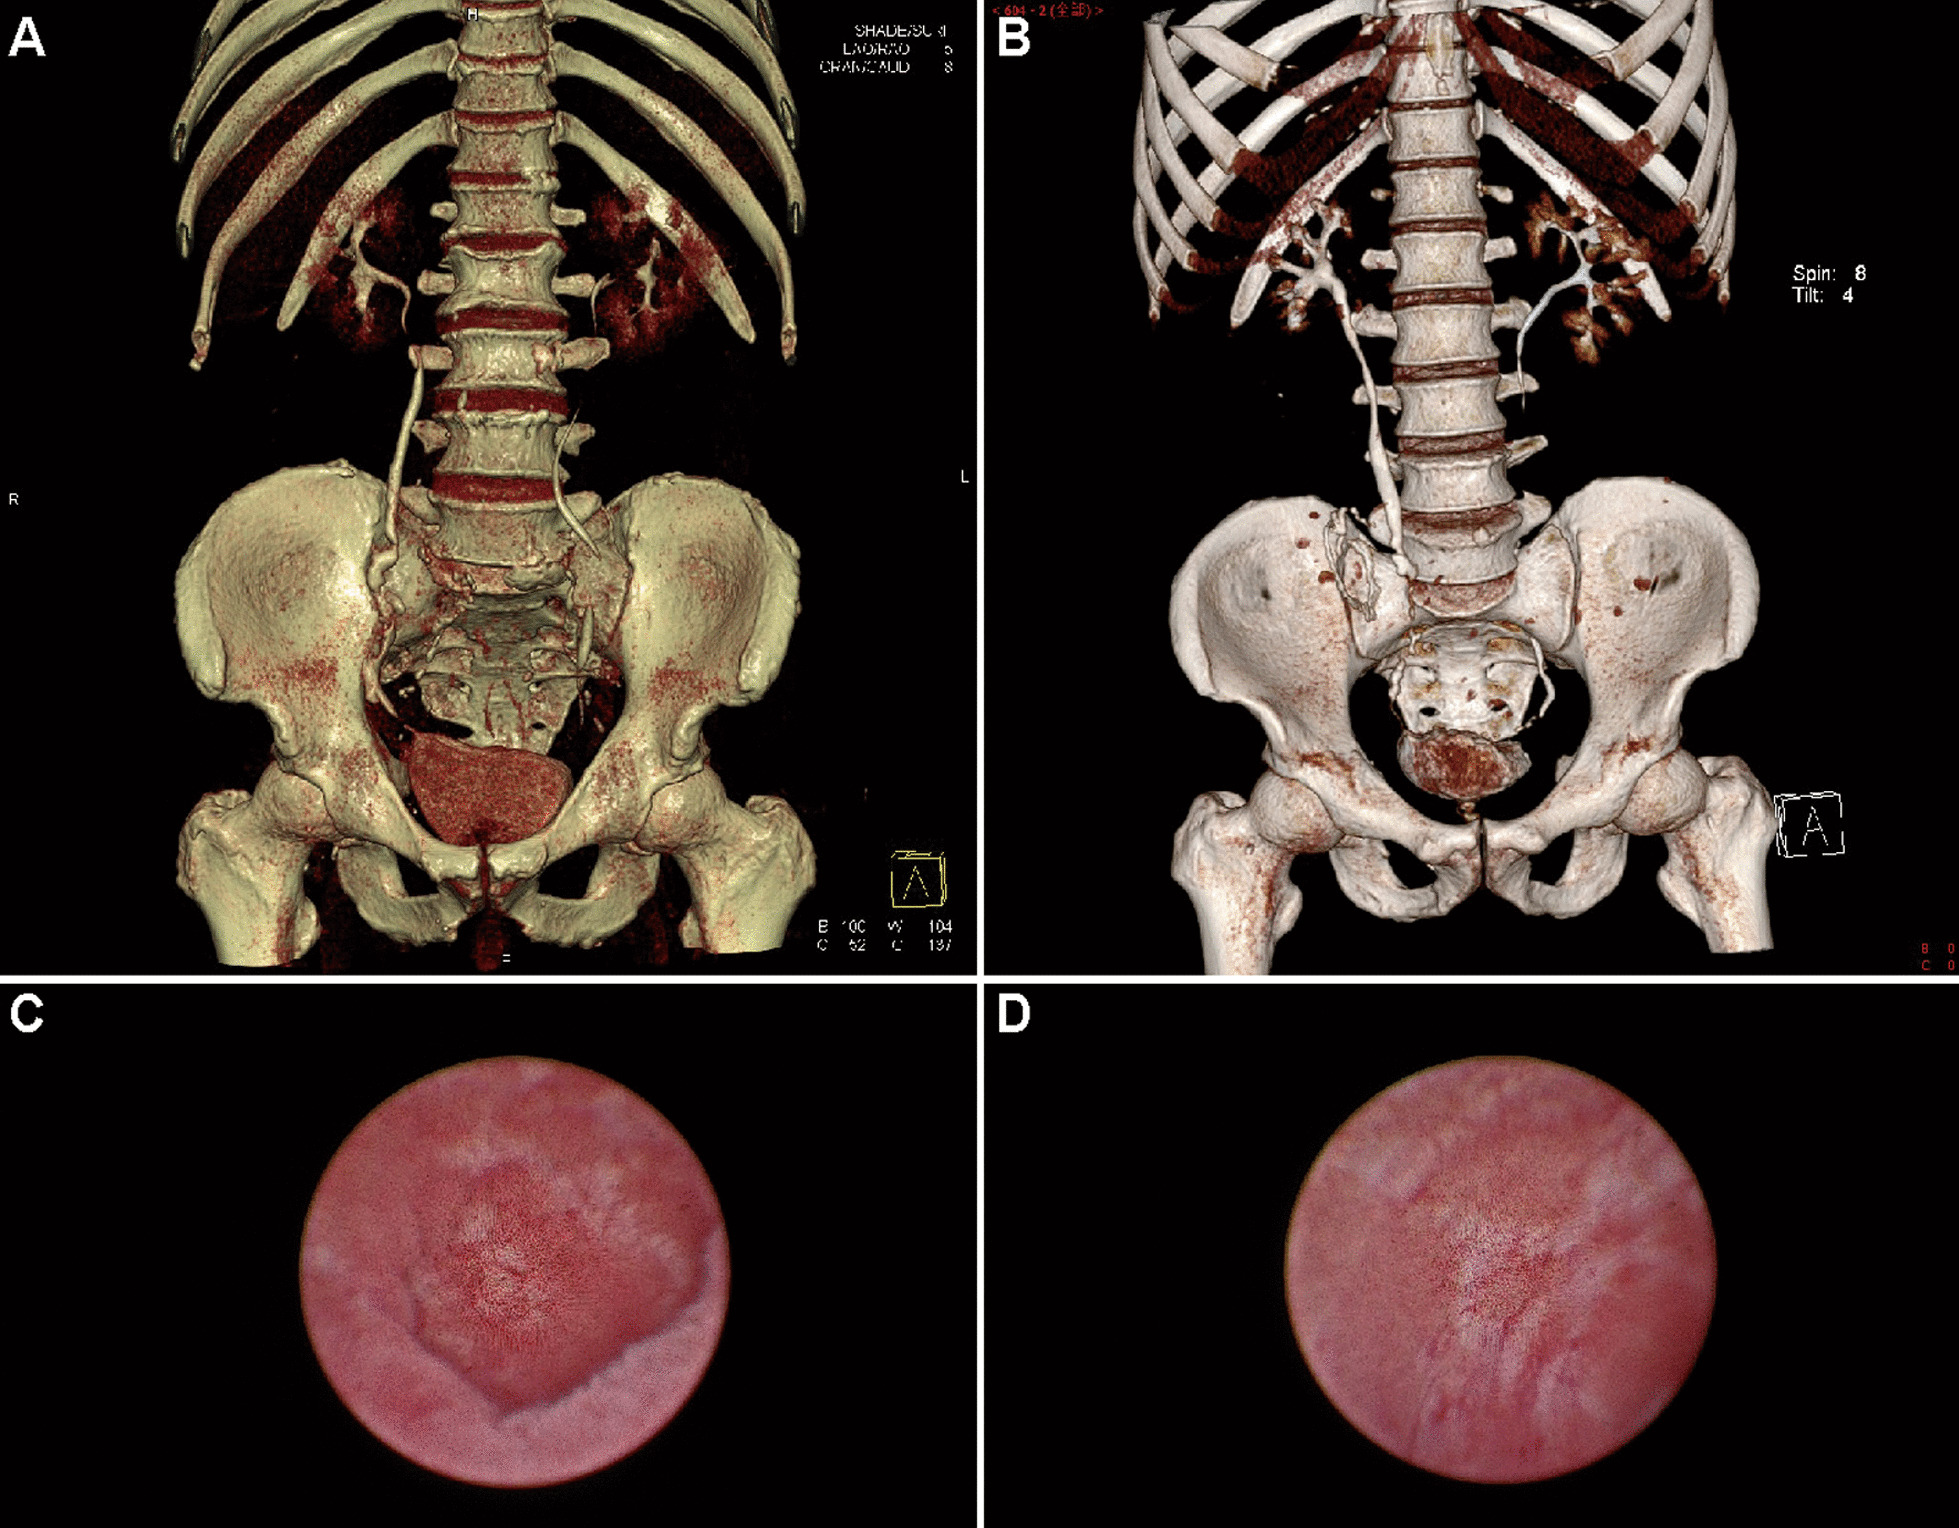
Images of patient 3 and 4. A CT urogram of patient 3 in month 36 post-surgery. B CT urogram of patient 4 in month 23 post-surgery. C Ileal mucosa regrowth of patient 3 in month 30 post-operation. D Ileal mucosa regrowth of patient 4 in month 18 post-operation
